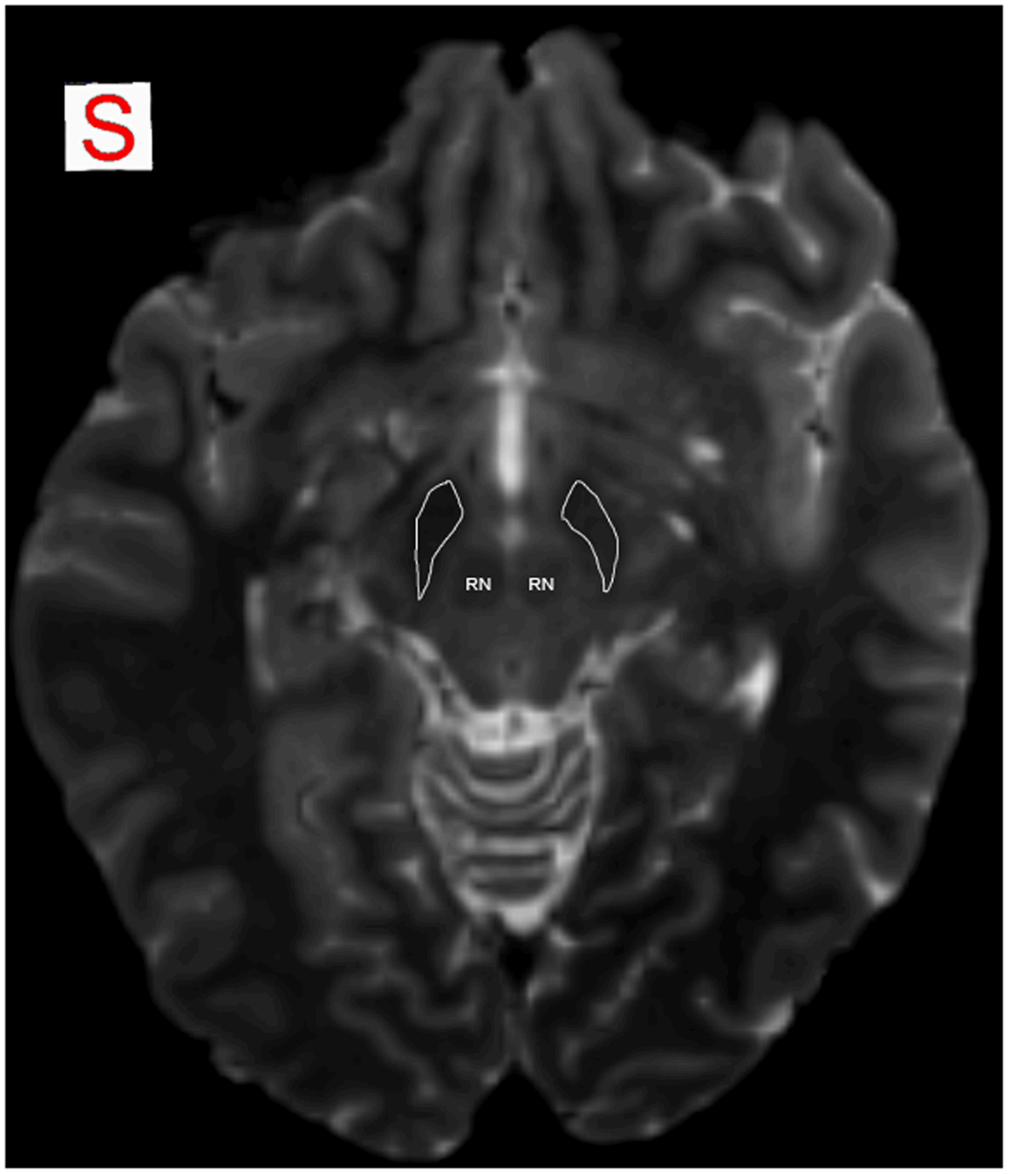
MRI identification of SN. SN is detectable as an hypointense region in axial plane in T2-weighted images, due to T2^*^ effect that allows a better visualization of the iron-loaded nuclei. Anatomical relations of SN with RN are well identifiable. RN, red nucleus; SN is encircled in white.
